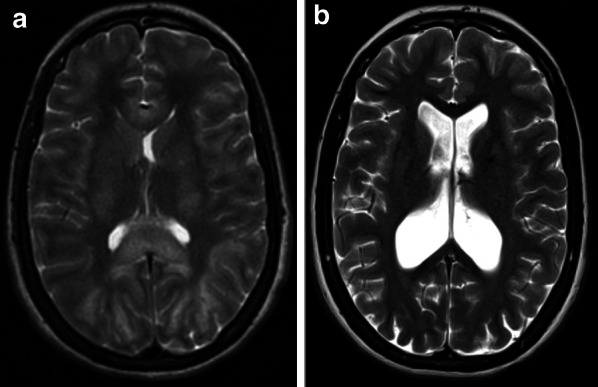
A case representing the long-term radiological consequences of CFE. T2 MRI images at the level of the basal ganglia of a 16-year-old polytraumatised female patient acquired on day 12 after hospital admission (a), and 16 months later (b). The long-term follow-up images show resolution of the subcortical and corpus callosum T2 hyperintensities, and dilation of the intergyral sulci, and the lateral ventricles indicative of cerebral atrophy (b)
